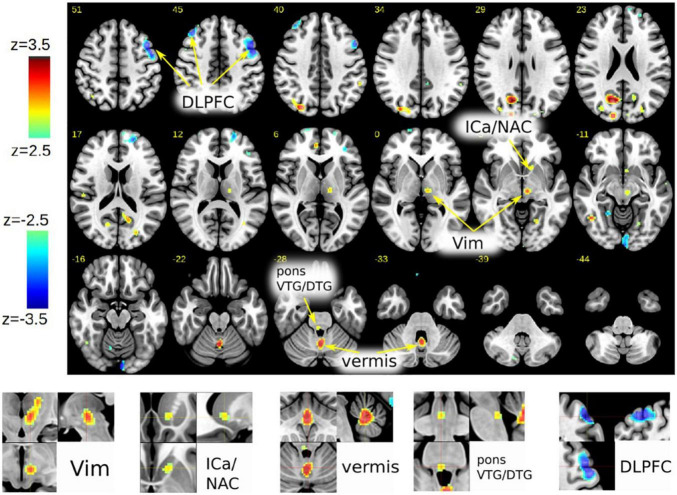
Case #1, FDG-PET activation pattern contrasting DBSon vs. DBSoff. Effects shown are related to initiation of DBS. Pattern description in text. (DLPFC, dorsolateral prefrontal cortex; ICa, anterior limb of internal capsule; NAC, nucleus accumbens; Vim, ventral intermediate nucleus of thalamus; VTG, ventral tegmental nucleus of Gudden; DTG, dorsal tegmental nucleus of Gudden.)
